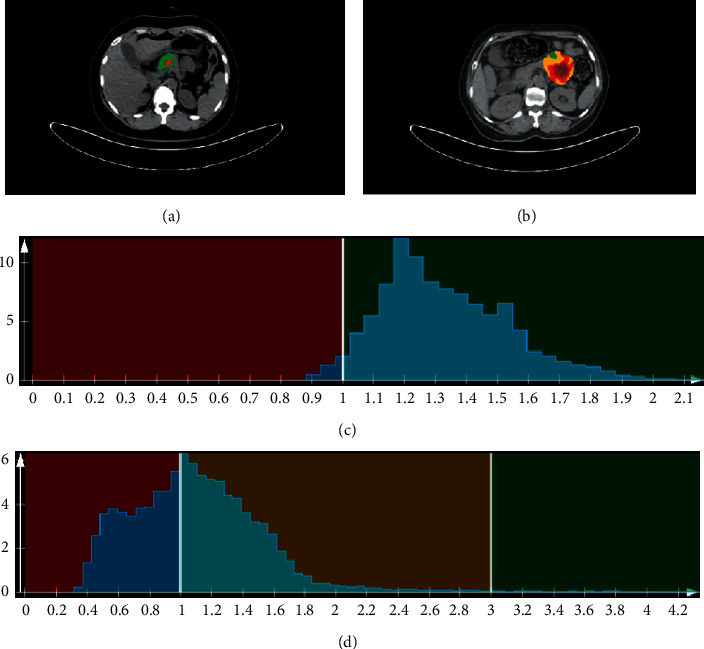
(a) PET/CT image of a 30-year-old female patient. The pathological result was SCNs. (b) PET/CT image of a 57-year-old female patient. The pathological result was MCNs. (c) and (d) are the VOI-based texture analysis histograms of PET image in (a) and (b), respectively. The histogram analysis showed skewness of each VOI as 0.612 and 1.811 in (c) and (d), respectively, and kurtosis as 0.168 and 6.979 in (c) and (d), respectively.
